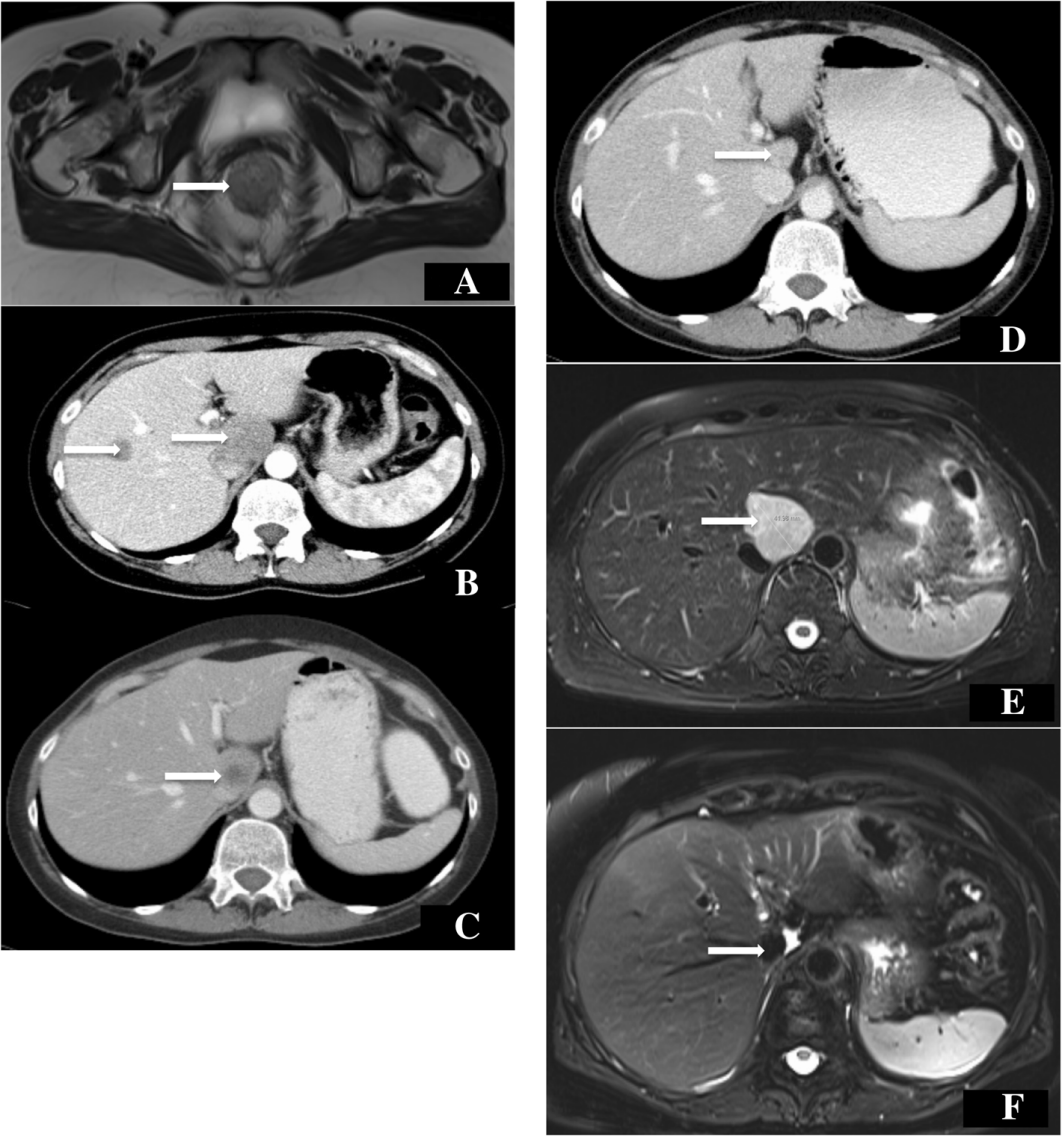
Deep seated, wall-penetrating rectal carcinoma (a, arrow, magnetic resonance imaging (MRI) with multiple bilateral liver metastases (b, arrow, computed tomography (CT) before treatment in April 2012. The patient reached partial remission after two cycles of cisplatin/irinotecan (c, residual metastasis in segment I with an arrow, CT) in June 2012 and complete remission after six cycles (d, arrow: no evidence of macroscopic metastasis in segment I, CT) in October 2012. View of segment I showing post-treatment recurrence in February 2013 (e, arrow, MRI) and status 5 years after stereotactic body radiotherapy and chemotherapy in February 2018 (f, no evidence of vital tumor: arrow, MRI)
